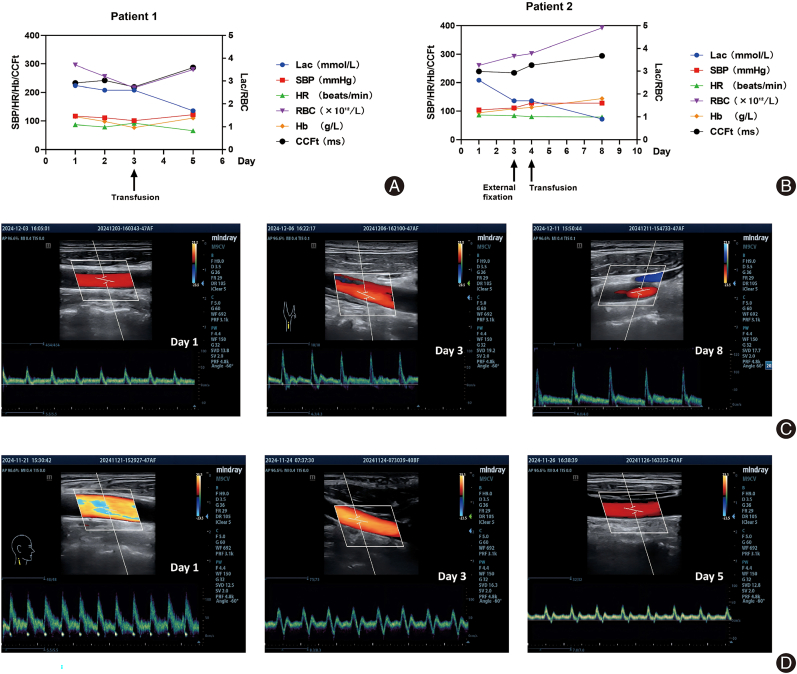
Dynamic monitoring of hemodynamic and metabolic indices and representative ultrasound images in patients with hemorrhagic shock during treatment. (A-B) Multi-parameter dynamic trend charts for 2 representative hemorrhagic shock patients during resuscitation, with key clinical interventions (e.g., transfusion, surgery) annotated along the timeline. The graphs display the time-course changes in SBP, HR, Hb, RBC, Lac, and CCFt. (A) Patient 1 (upper gastrointestinal bleeding): On Days 1, 3, and 5 of admission, following hemostasis and transfusion, Hb and RBC recovered, lactate cleared, and CCFt significantly prolonged (recovering from a nadir of 219.0 ms to 286.9 ms). (B) Patient 2 (open fracture): From Day 1 to Day 8 of admission, following surgery and transfusion support, CCFt showed an improving trend consistent with the rise in Hb and the decline in Lac, eventually restoring to 293.6 ms. (C-D) Comparison of carotid artery pulsed-wave Doppler spectral morphology between Patient 1 (C) and Patient 2 (D) at different treatment stages. During the shock stage, the spectrum presents a narrow and sharp systolic peak with a steep ascending limb and a rapidly descending limb, corresponding to a significant shortening of the CCFt. With the recovery of effective circulating blood volume, the spectral waveform broadens markedly, and CCFt prolongs to the normal range. SBP: systolic blood pressure; HR: heart rate; Hb: Hemoglobin; RBC: red blood cell count; CCFt: carotid corrected flow time; SI: shock index.
